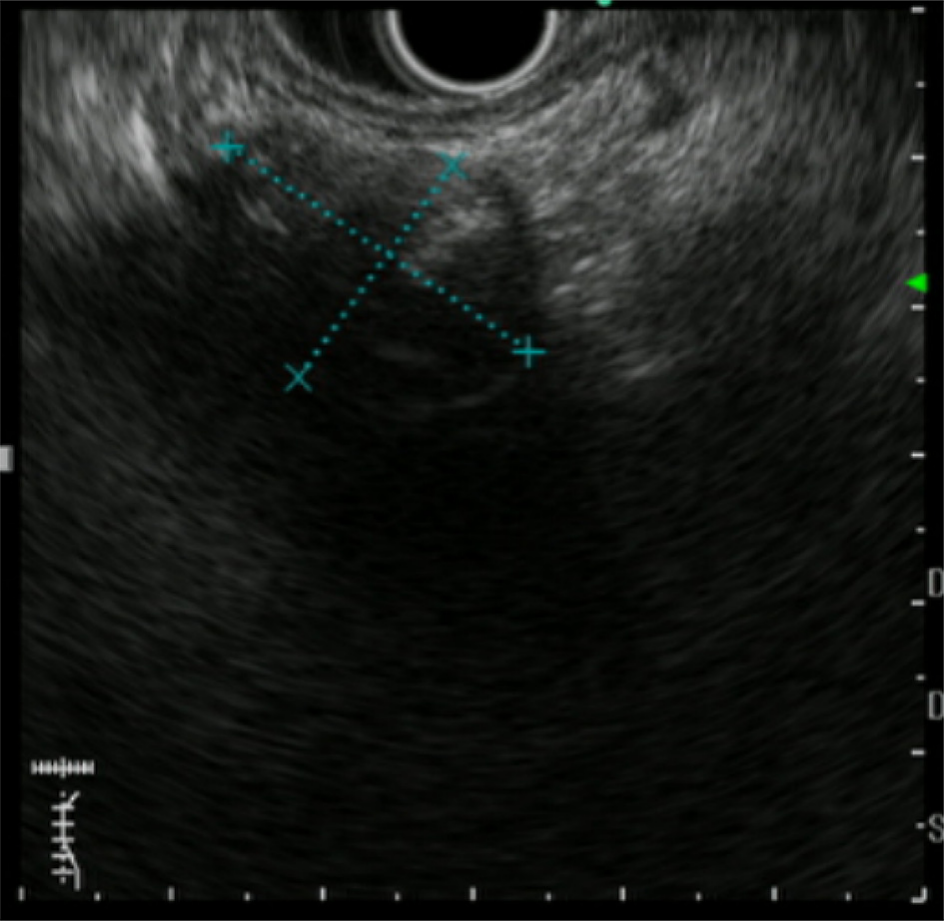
Findings on endoscopic ultrasonography. The mass is a hypoechoic lesion containing a high spot with an acoustic shadow.
